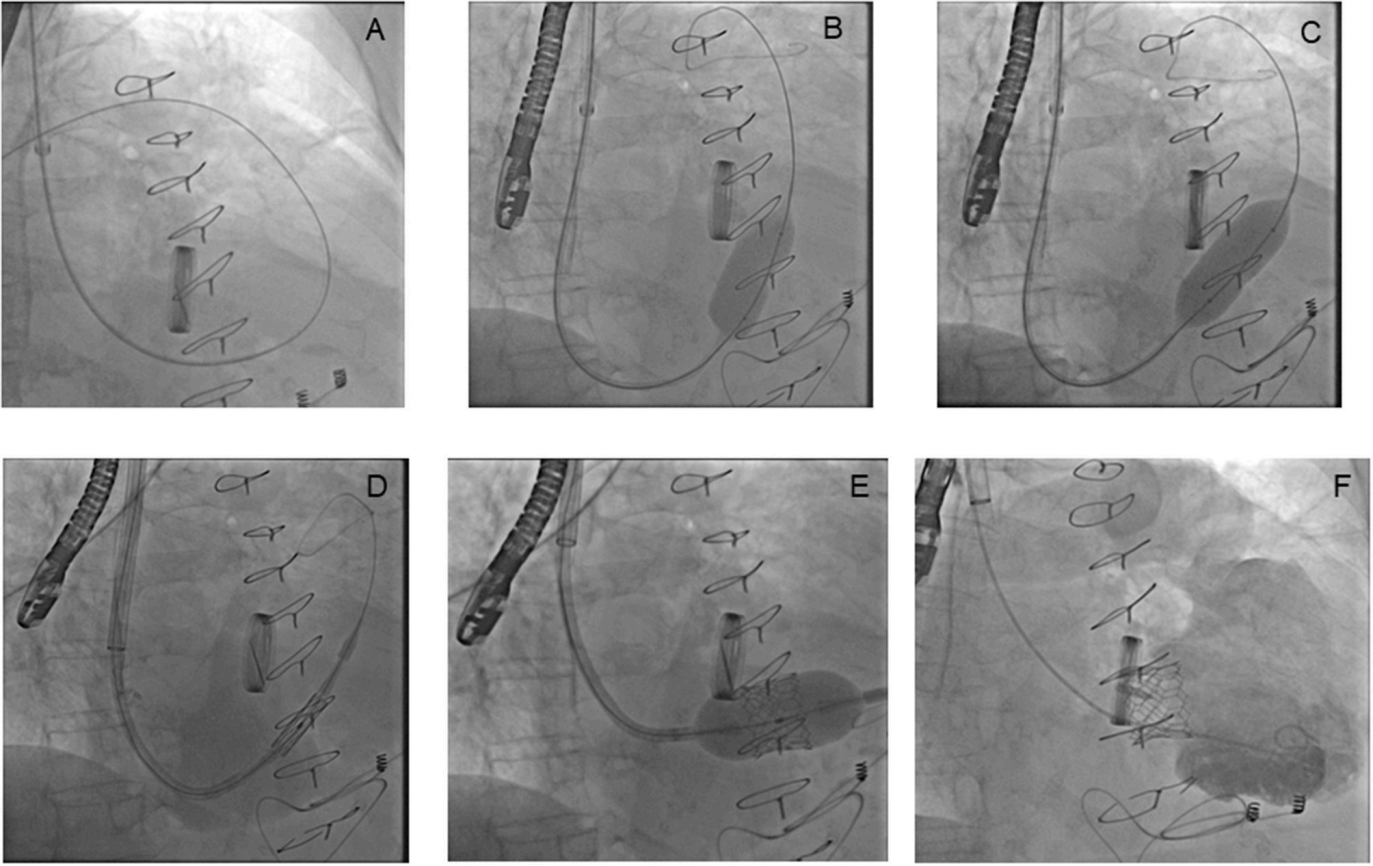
The implantation of an aortic balloon expandable biological valve into a degenerated tricuspid biological prosthesis. A Swan Ganz catheter with an angiographic 0.035' wire crosses the tricuspid degenerated prosthesis (A). A balloon valvuloplasty is performed, even if no mandatory in valve in valve procedure. The first balloon inflation shows a residual stenosis (B), the second inflation shows a successful balloon expansion. The valvuloplasty is also performed to confirm the degenerated bioprosthesis size (C). An aortic balloon expandable biological valve, a device normally used for aortic valve replacement, crosses the tricuspid degenerated prosthesis (D). The guidewire is placed in right ventricle to achieve a perfect alignment between transcatheter valve and degenerated bioprosthesis despite the horizontal right chamber axis. The valve is deployed using a 3D transesophageal echocardiography (TEE) reconstruction and right atrial angiography to control the release (E). The final right ventricular angiography shows no residual regurgitation after valve in valve implantation (F).
